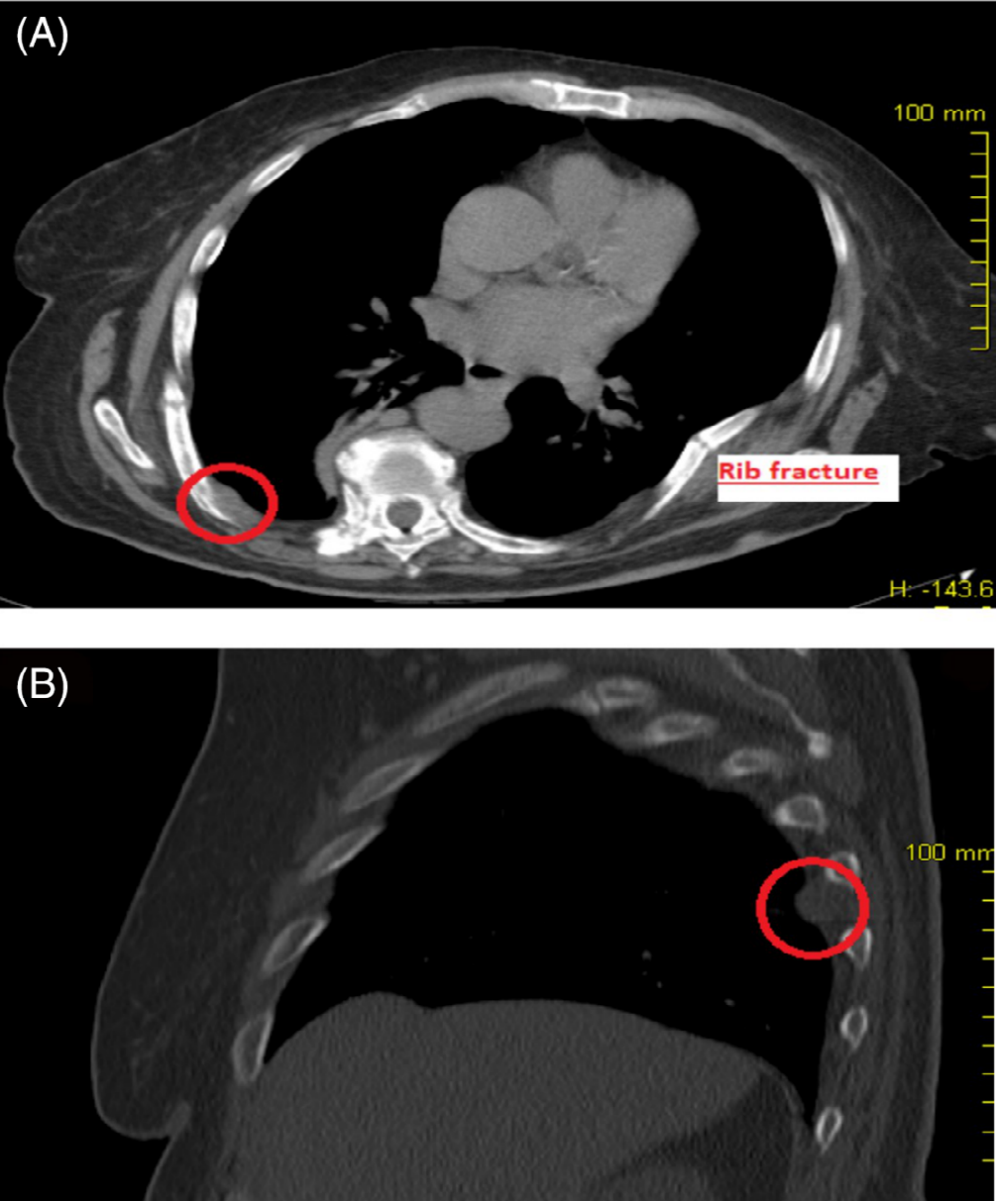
(A, B) CT images of intrathoracic phosphaturic mesenchymal tumor and rib fracture
